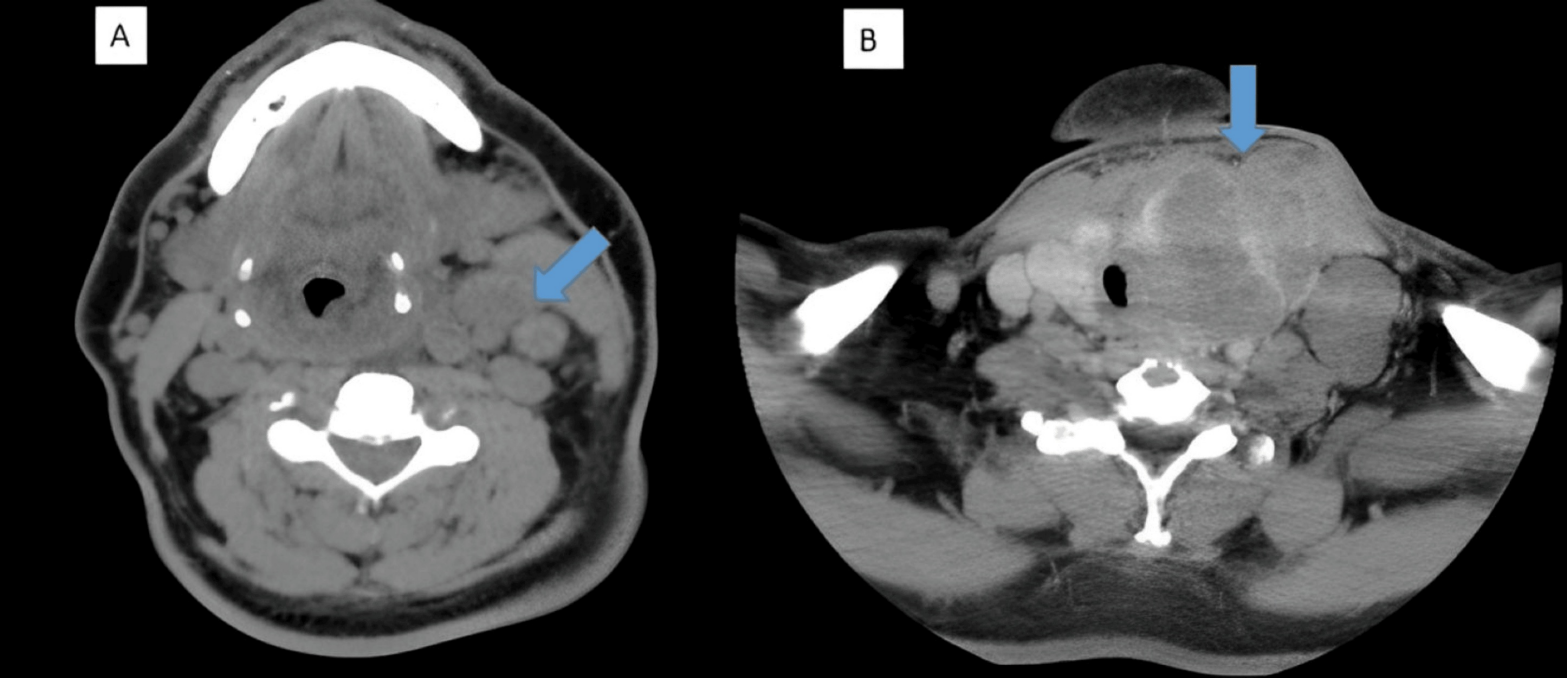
Cervical-thoracic CT (A) Axial section showing left jugulo-carotid adenopathy. (B) Axial section revealing a large tumor in the left thyroid lobe, extending into the thyroid isthmus and invading the left sternocleidomastoid muscle anteriorly and toward the right. There is also posterior vertebral invasion with lytic destruction of the C7 vertebral body. This mass causes medial displacement and compression of the trachea, along with lateral compression of the left jugular vein. Multiple left supraclavicular lymphadenopathies are also observed.
